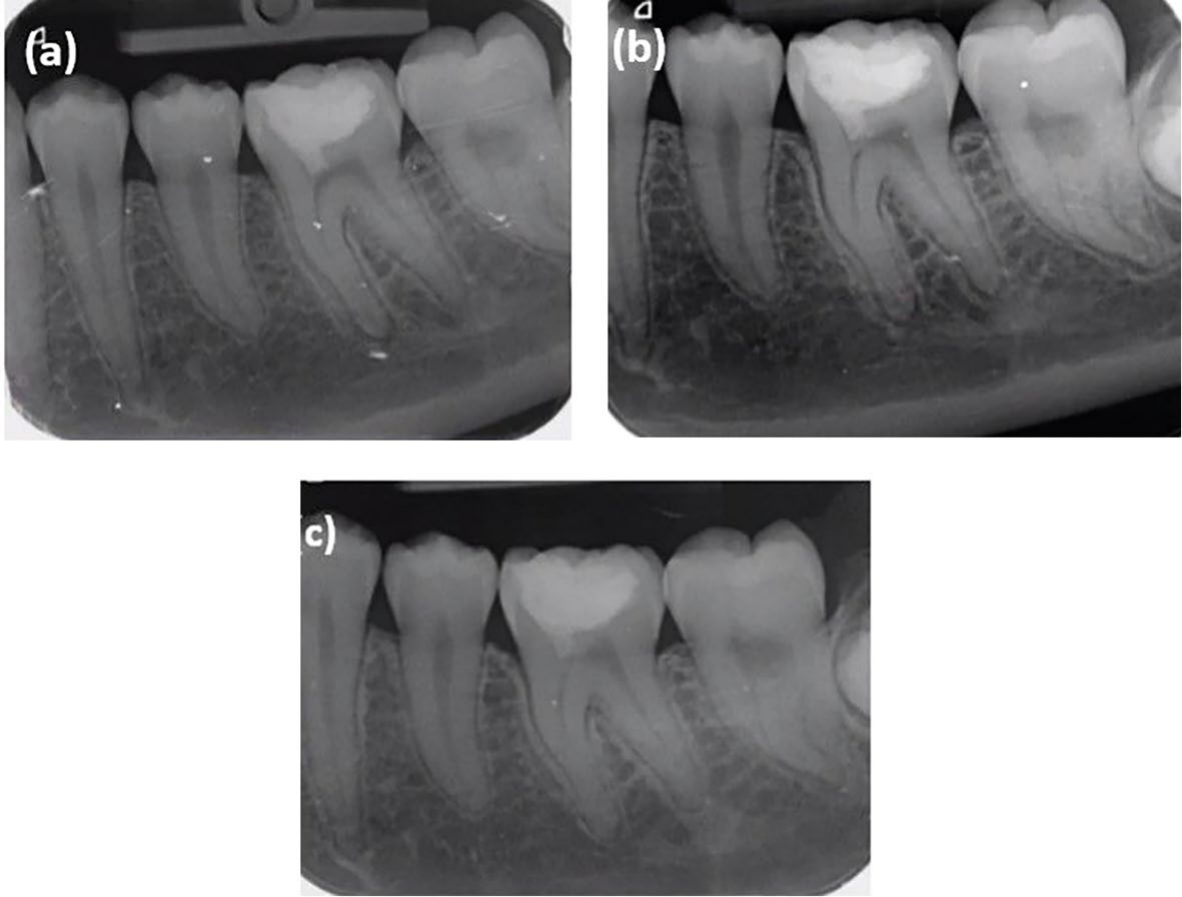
Post-operative radiographs. a) At baseline. b) At six months review. c) At two years and two months review
